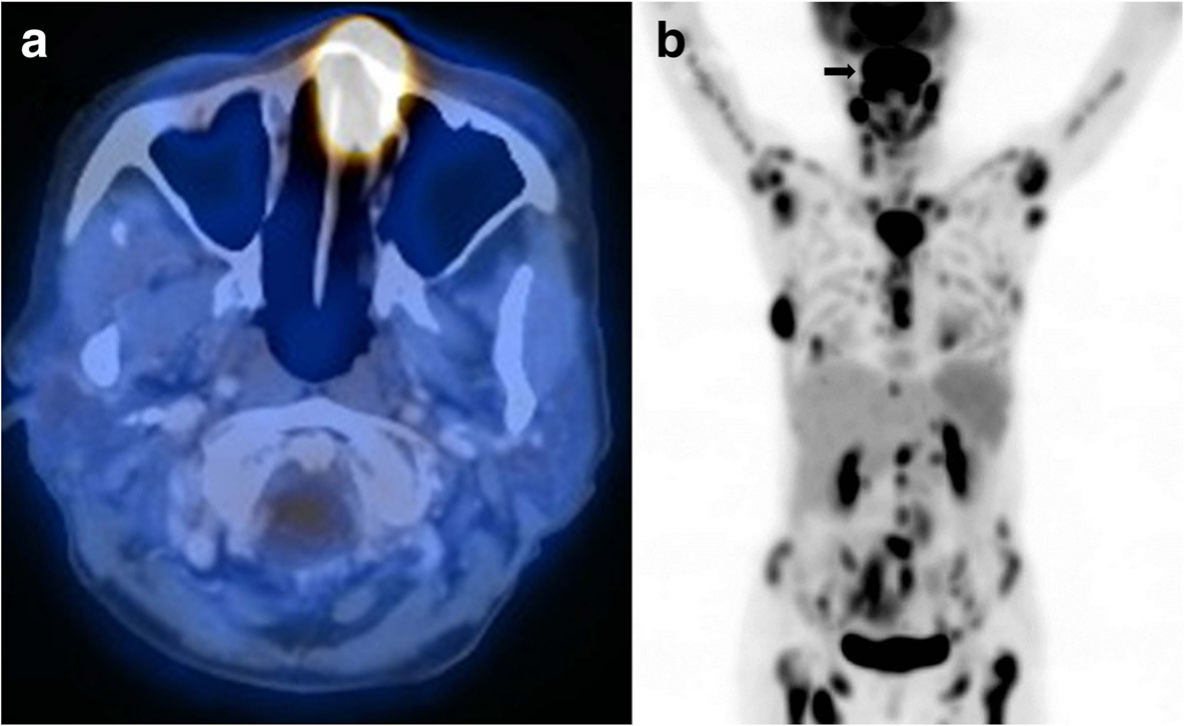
Positron emission tomography computed tomography of NK/T-cell lymphomas. a Nasal lesion that shows avidity for 18F-fluorodeoxyglucose. b Disseminated disease. Note the large nasal tumor (*arrow*) and multiple hypermetabolic lesions in other anatomical sites
